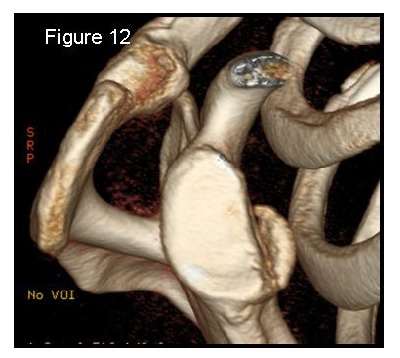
3D-CT reconstruction glenoid en face view to assess and quantify the glenoid bone loss. 62x56mm (150 x 150 DPI).
